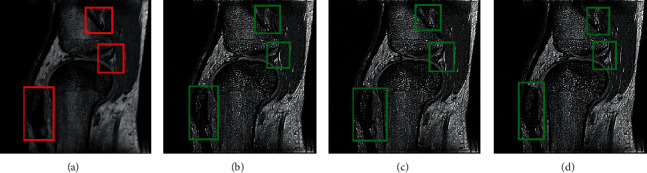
The sharpen results of “Knee MRI medical image”: (a) source image, (b) sharpen image by Laplacian filter, (c) sharpen image by unsharp masking, and (d) LF + DFT sharpen image.
